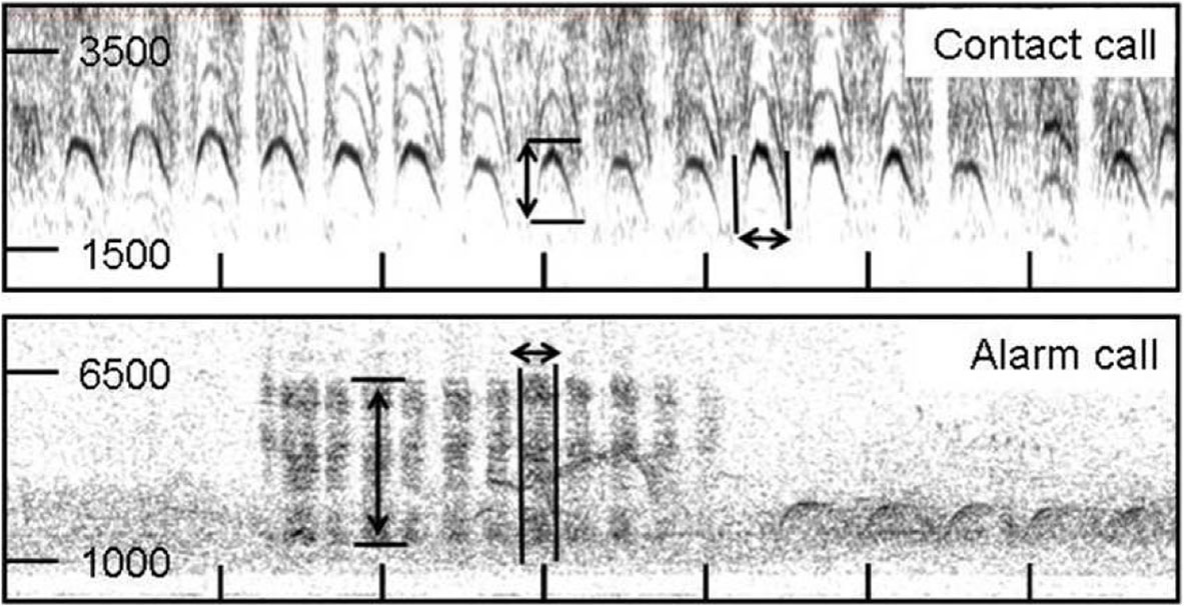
Typical sonogram of an alarm call (above) and a contact call (below) of *Turdoides hindei*, recorded south of Kitui. The following call characteristics were analysed: highest and lowest frequency (circles), and frequency range and length of single calls (arrows)
